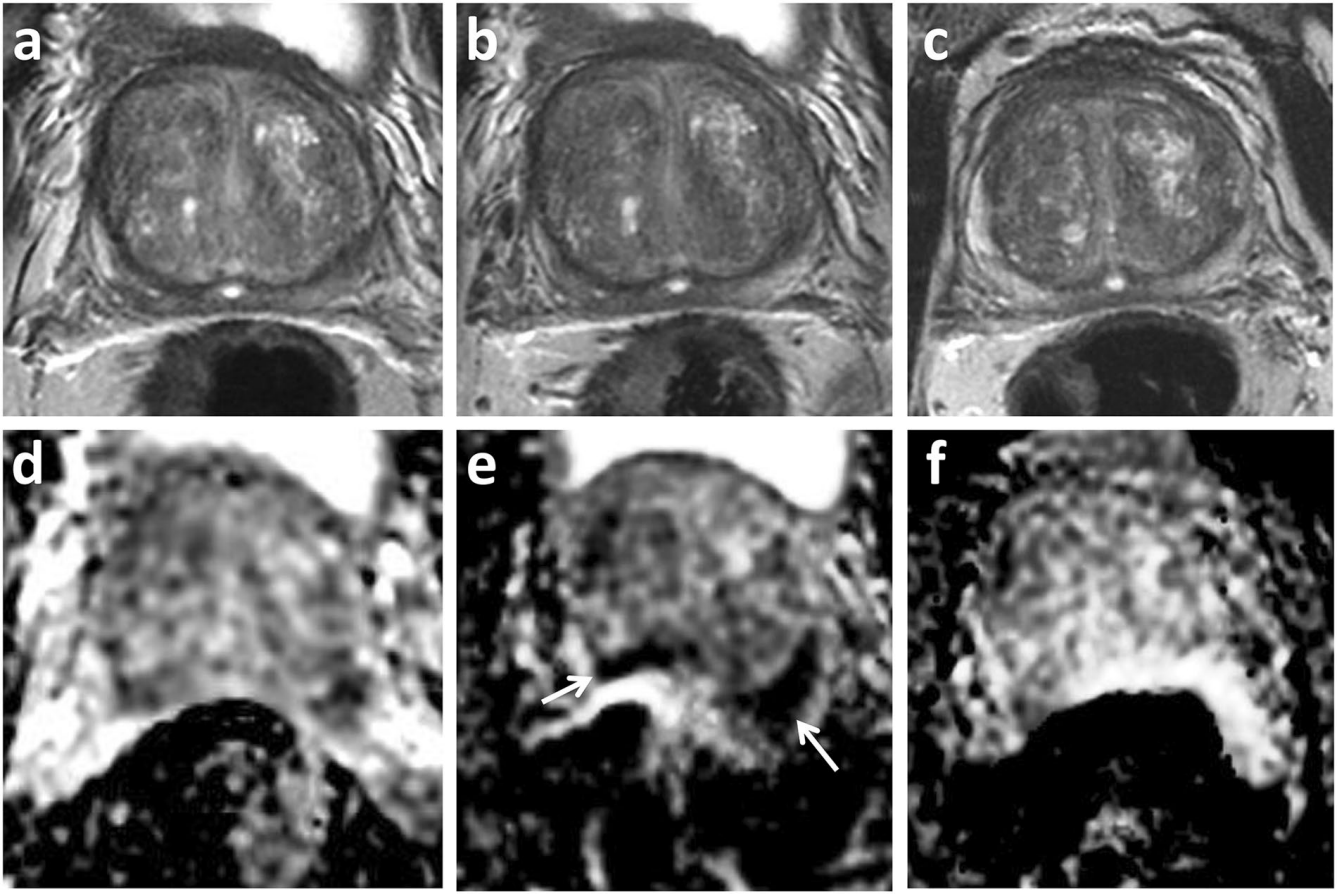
Distortion artefact in active surveillance. 66 year old patient on active surveillance. PSA 6.02 ng/ml at enrolment MRI 2014 (a,d), and stable at follow-up imaging in 2015 (b,e) and 2016 (c,f). Top row T2-weighted imaging, bottom row ADC maps. 2015 study included within current cohort shows posterior warping with apparent new and marked restricted diffusion (arrow), this is artefactual and not present on subsequent follow-up imaging.
